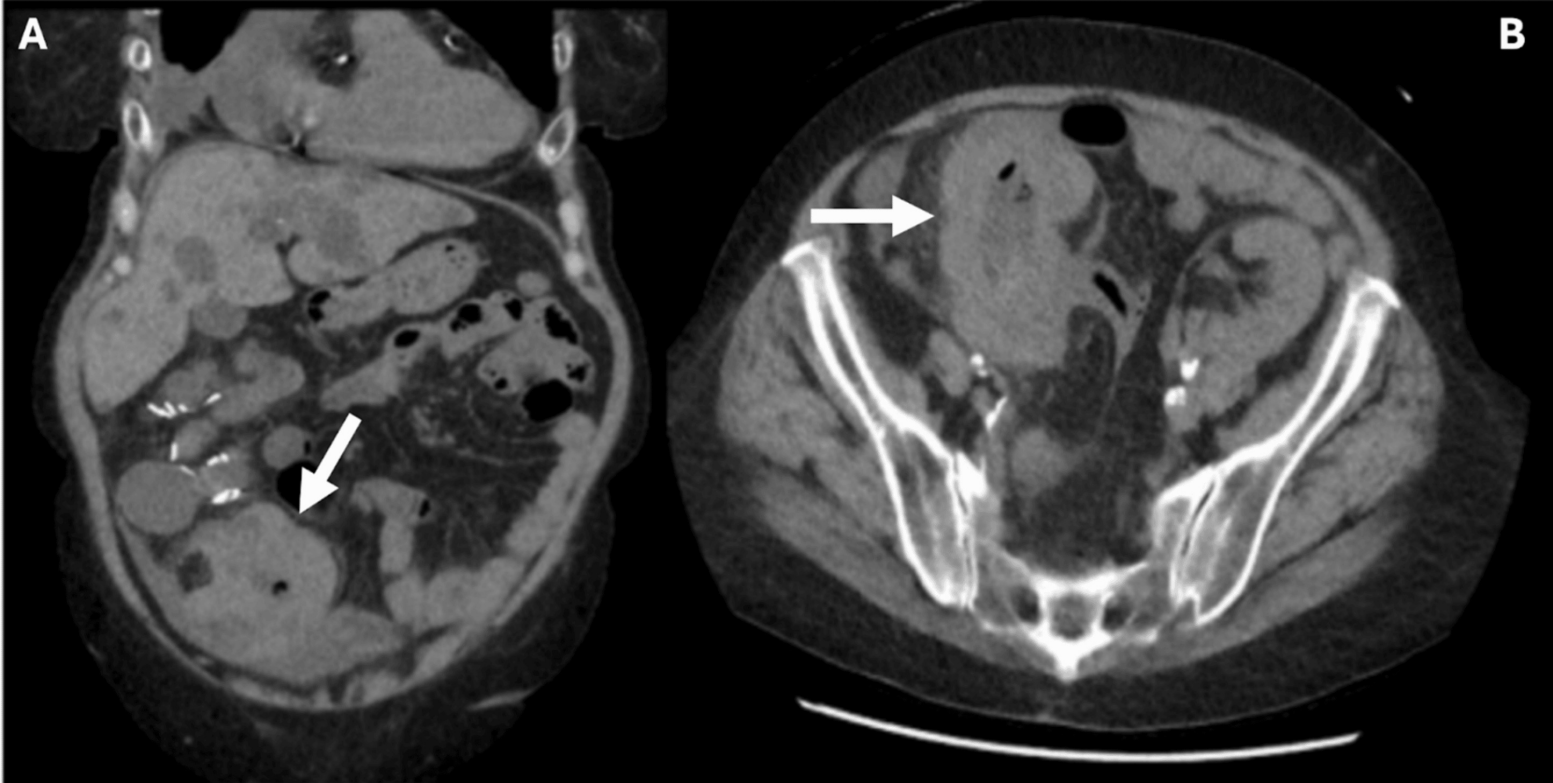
(A and B) Contrast-enhanced CT of the abdomen and pelvis (coronal and axial) demonstrating marked mural thickening of the cecum and ascending colon with pericolonic stranding.
